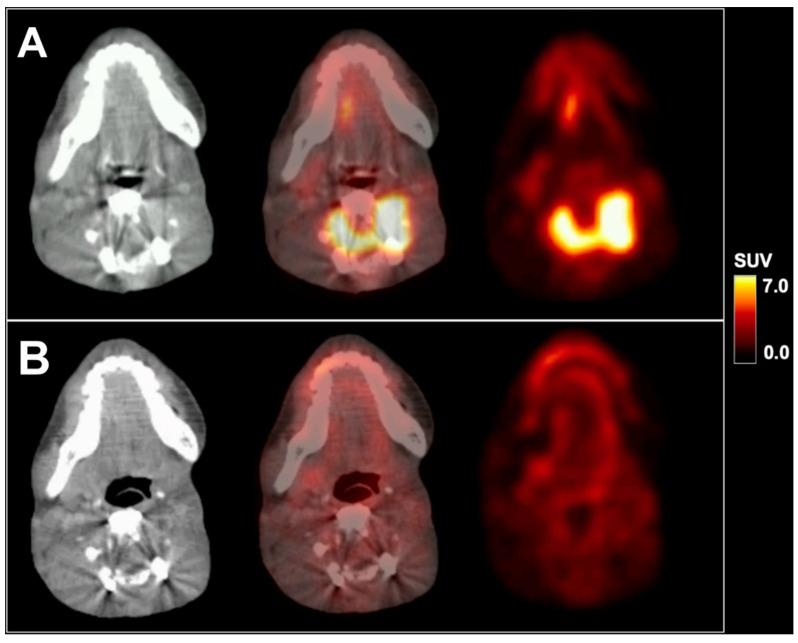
^18^F-FDG PET/CT in axial planes of a metastasis in the cervical spine before (A) and after radiochemotherapy (B). SUV = standardized uptake value.
